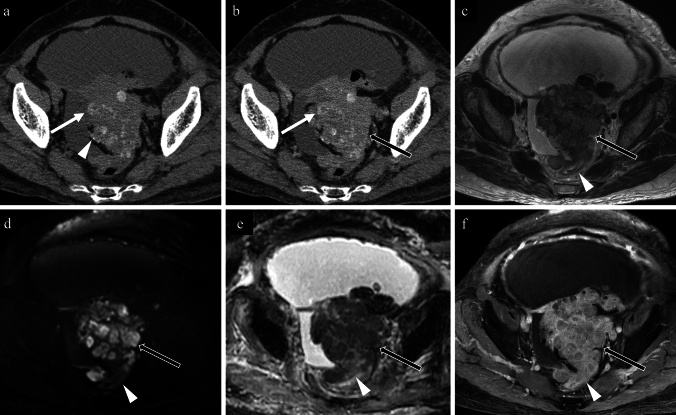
73-year-old woman with a high-grade serous carcinoma of the left fallopian tube. a non-contrast CT; b contrast-enhanced CT; c axial T2-weighted image; d axial diffusion-weighted image; e axial apparent diffusion coefficient map; f axial contrast-enhanced T1-weighted image. A lobulated mass in the sigmoid colon fossa is observed, accompanied by diffuse amorphous calcifications (a; white arrow) and punctate calcifications (a; white arrowhead) on non-contrast CT. Contrast-enhanced CT shows faint enhancement of the entire mass (b; black arrow), and it is difficult to determine the presence of calcification only on contrast-enhanced CT (b; white arrow). T2-weighted image demonstrates heterogeneous signal intensities (c; black arrow). Diffusion-weighted image shows strong but heterogeneous diffusion restriction (b and e; black arrows). Postcontrast T1-weighted image shows moderate enhancement (f; black arrow). Infiltration from the rectum is also observed (c–f; arrowheads). Pathological findings confirmed the diagnosis of high-grade serous carcinoma originating from the left fallopian tube
